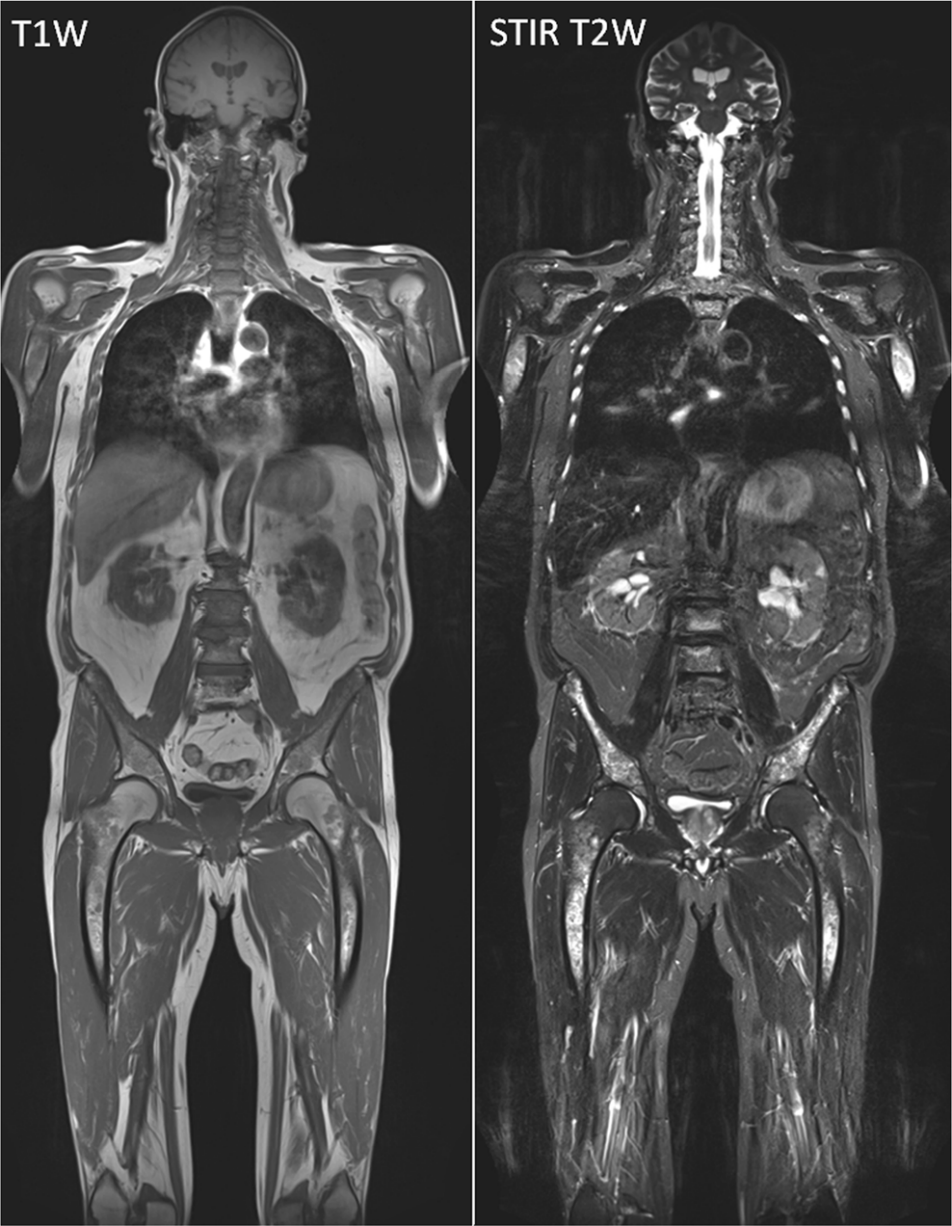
Coronal T1-weighted (*left*) and T2-weighted STIR (*right*) coronal whole body MR images displaying a diffuse marrow infiltration in the spine, pelvis, femora, humeri, ribs and scapulae. Lesions appear hypointense on T1-weighted images and hyperintense on the STIR images. Remark the good contrast resolution of STIR images in revealing infiltration of the ribs: ‘white ribs sign’
